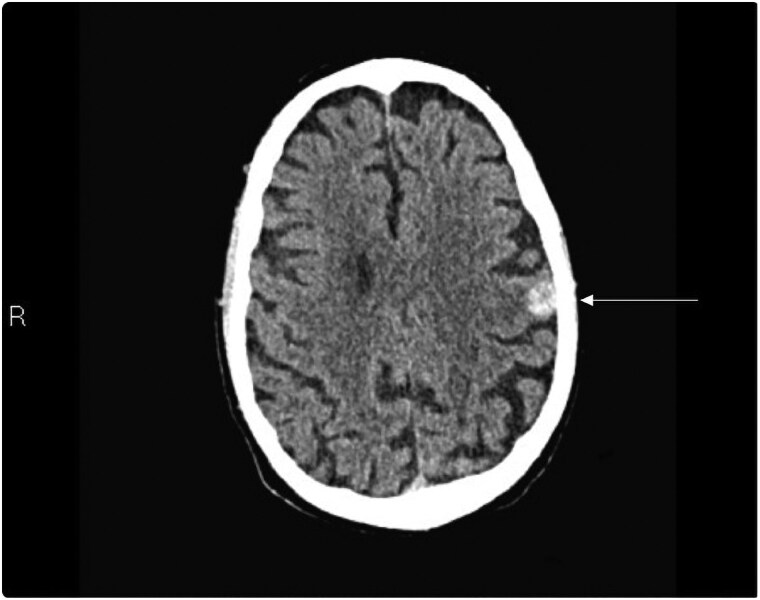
Non-contrast computed tomography scan axial images of the head demonstrating an acute haemorrhage in the left parietal lobe (white arrow).
